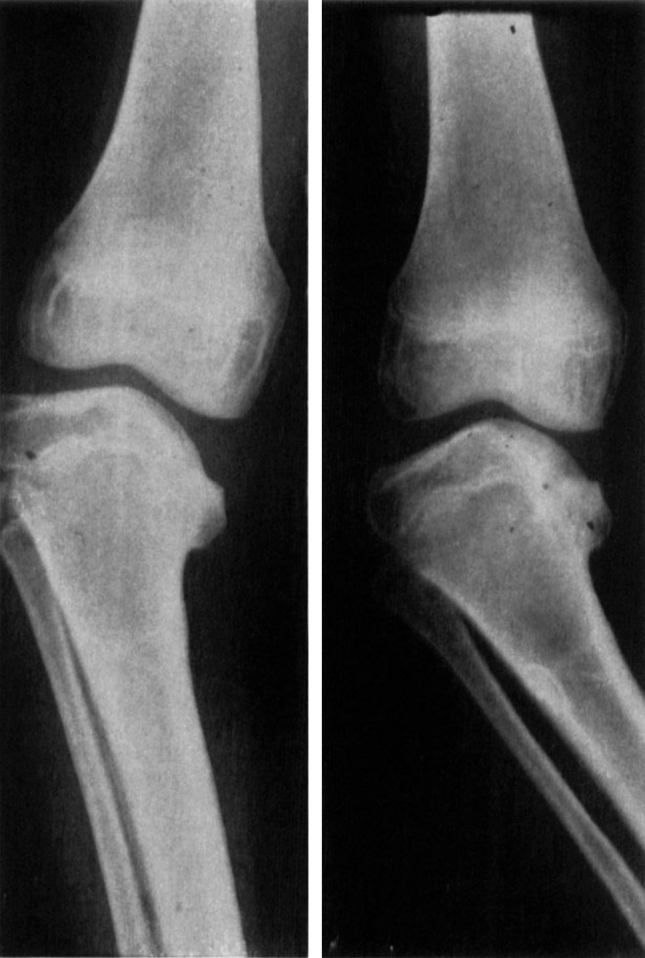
Preoperative radiographs, without weight-bearing (left). During weight-bearing (right) varus is increased because the defective medial condyle provides poor support for the femoral condyle. Reprinted from Støren (1969).
